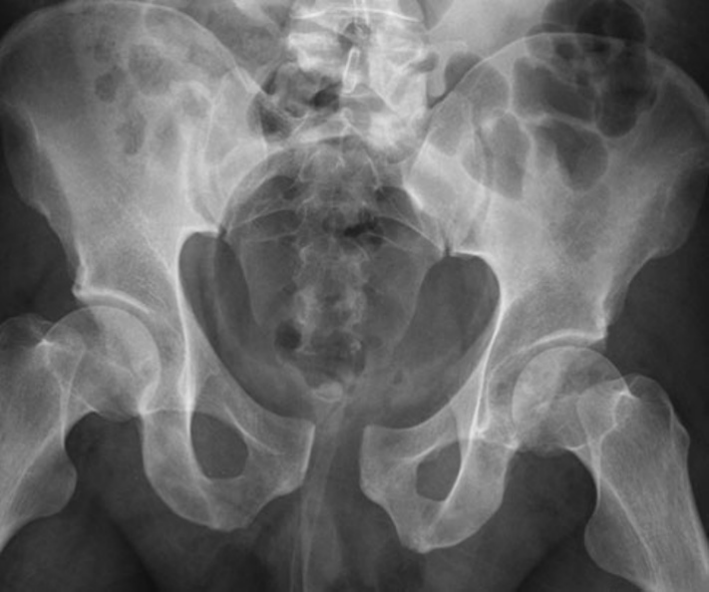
Initial pelvic injuries seen on an anteroposterior pelvic radiograph in case 2 include pubic symphysis diastasis, right sacroiliac diastasis and right sacral ala fracture
